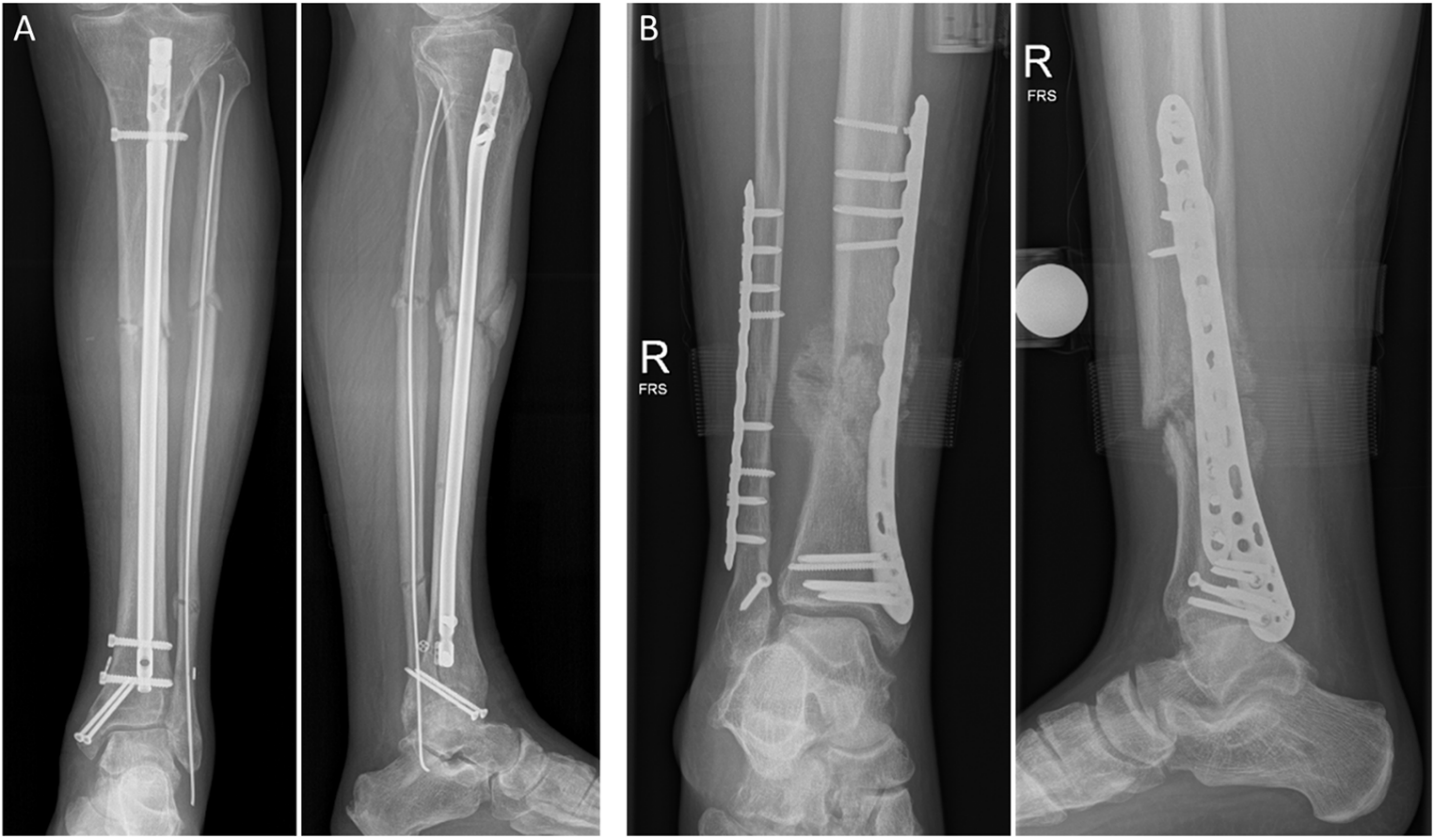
Radiographs of two patients with nonunion. (A) A 71-year-old man 140 days after surgery. (B) A 28-year-old man 125 days after surgery. Note the untreated syndesmotic diastasis that has likely contributed to the nonunion development.
